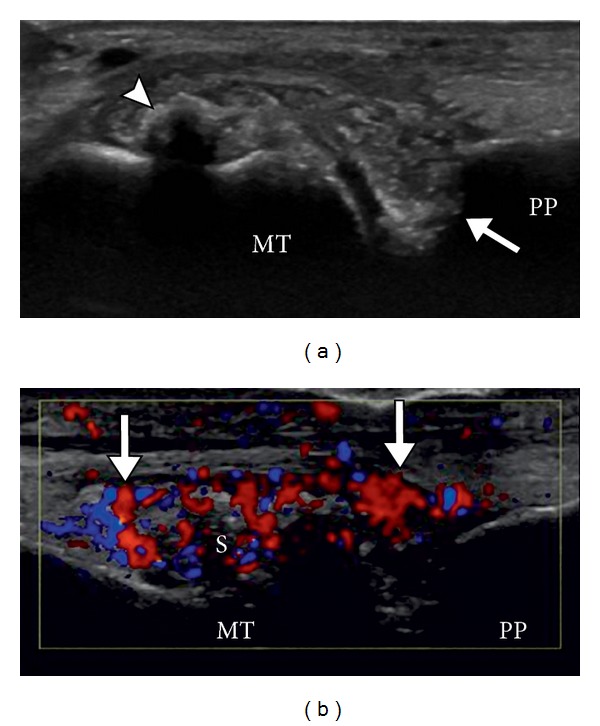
Gout with synovitis. Longitudinal US images of the 1st MTP joint without (a) and with (b) color Doppler show calcified, shadowing tophus (arrowhead) and adjacent heterogeneous soft tissue with associated hyperemia on color doppler imaging, consistent with synovial proliferation. Note the erosions at base of proximal phalanx (arrow).
